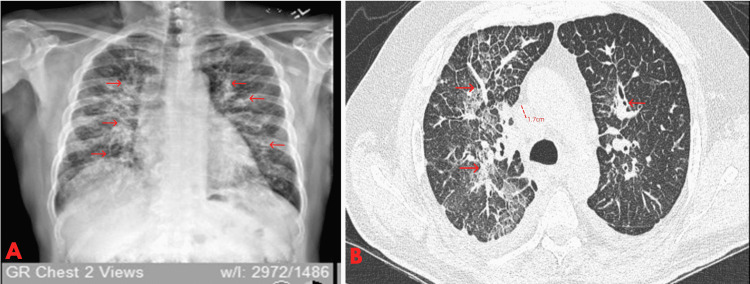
Chest X-ray and CT findings. A. Chest X-ray denoting diffuse bilateral patchy/heterogeneous opacities with prominent interstitial markings. B. Chest CT showing severe diffuse bilateral septal line thickening with patchy ground-glass opacity. Diffuse severe peribronchial thickening. Multifocal thoracic lymphadenopathy measuring up to 1.7 cm in size.
